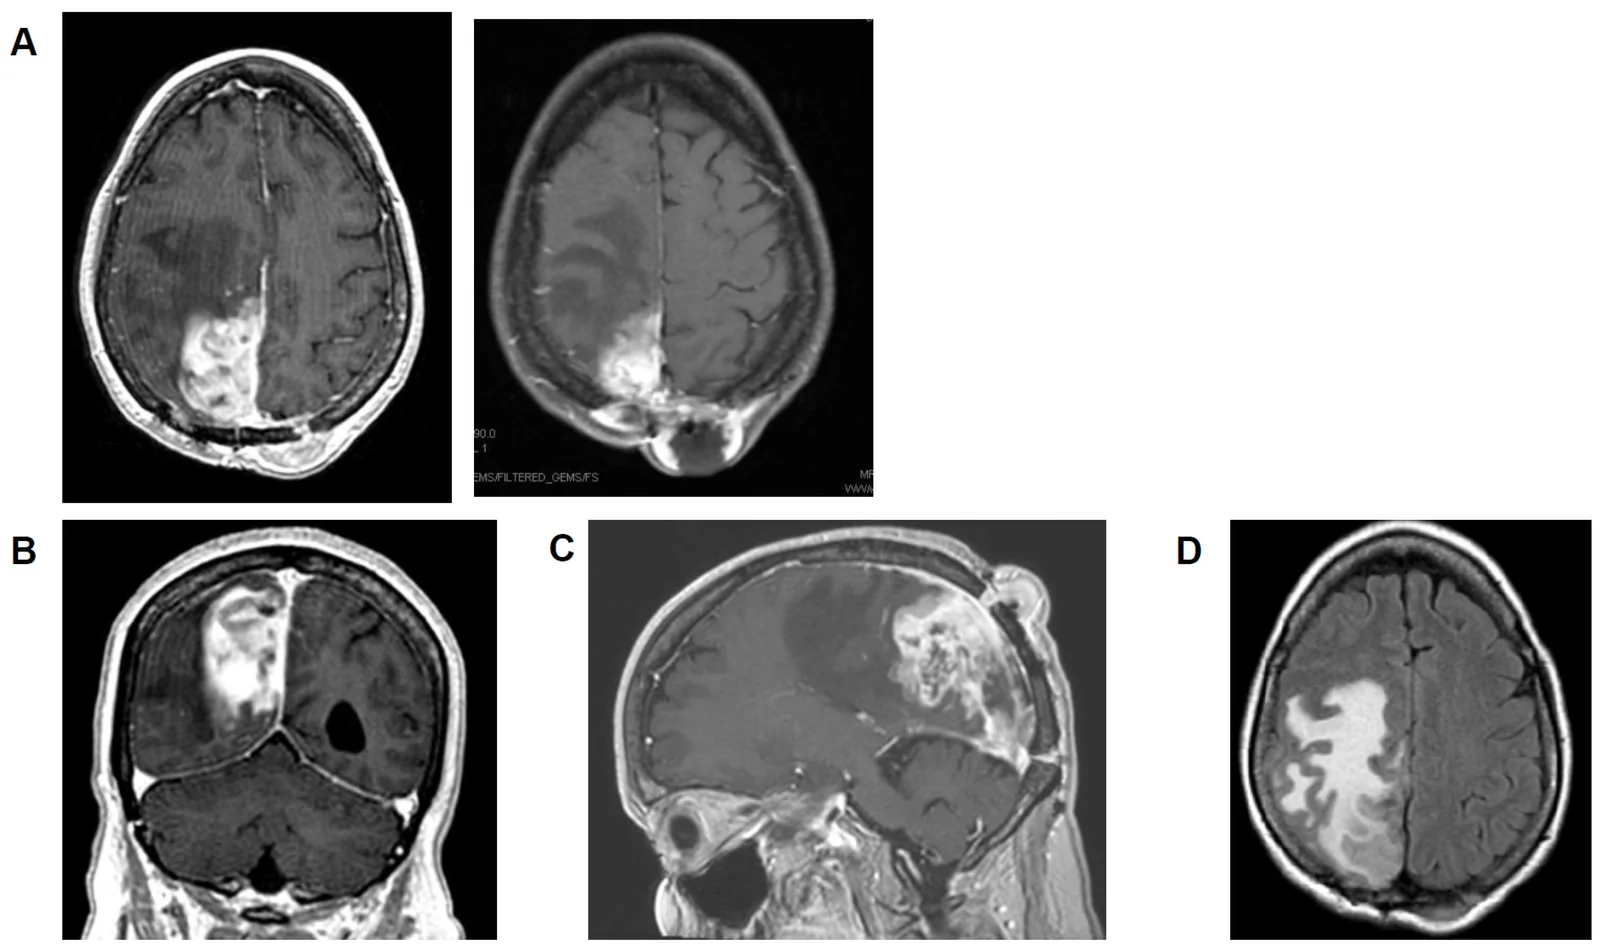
A 58 y/o female with BRAF mutant melanoma treated with two previous resections and two SRS treatments. She re-presented with headaches and left leg weakness. T1-weighted MRI with contrast (axial (A); coronal (B); sagittal (C)) showed a complex intracranial and extracranial re-recurrence involving the previous resection cavity, the adjacent calvarium and scalp. T2-weighted axial image (D) showed significant associated cerebral edema. She underwent re-resection followed by adjuvant WBRT.
